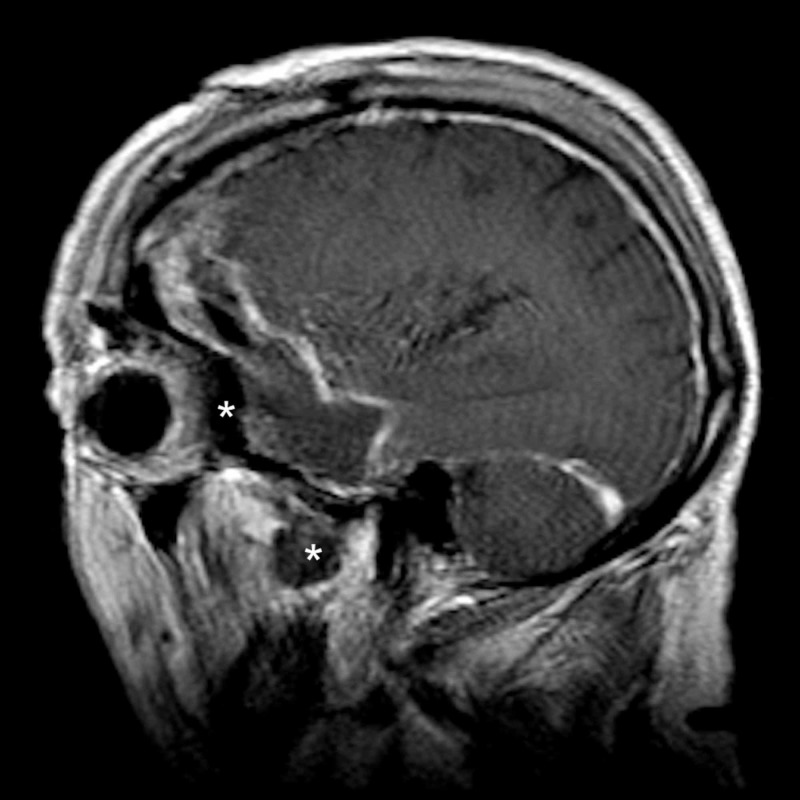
Postoperative sagittal MRI demonstrating resection of the intraorbital and intramuscular tumor component * = Areas of prior tumor invasion of the lateral orbit and temporalis muscle MRI: magnetic resonance imaging
